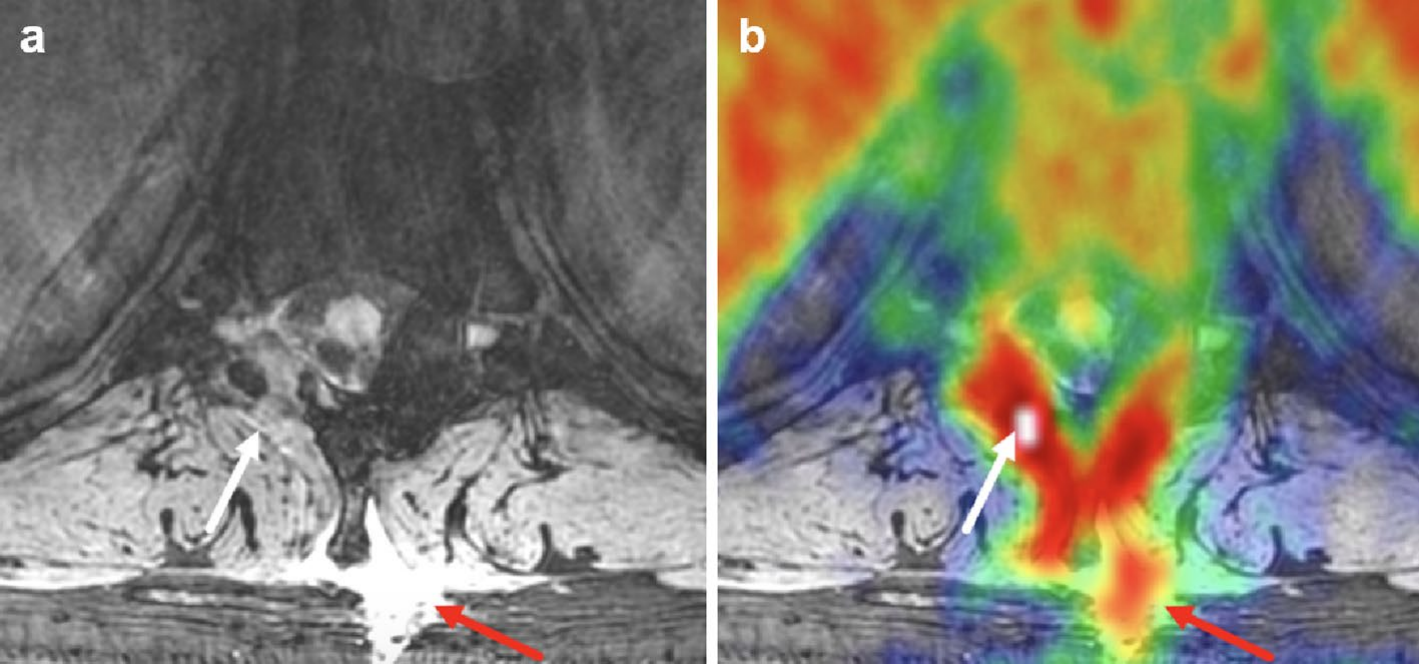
PET/MRI showing abnormalities at the level T10, especially at the site of a right-sided laminotomy, in patient with suspected cerebrospinal fluid leak. Axial DESS MRI (a) at T10 level of Patient 6 shows right-sided laminotomy (white arrow) with fluid collection (red arrow). Increased [18F]FDG uptake (white arrow, SUV_max_ = 4.6) is visible on PET/MRI (b) in areas corresponding to osseous tissue and neuroforamina. In addition, focal fluid collection is observed around the spinous process, which also has increased [18F]FDG uptake (red arrow, SUV_max_ = 2.6).
